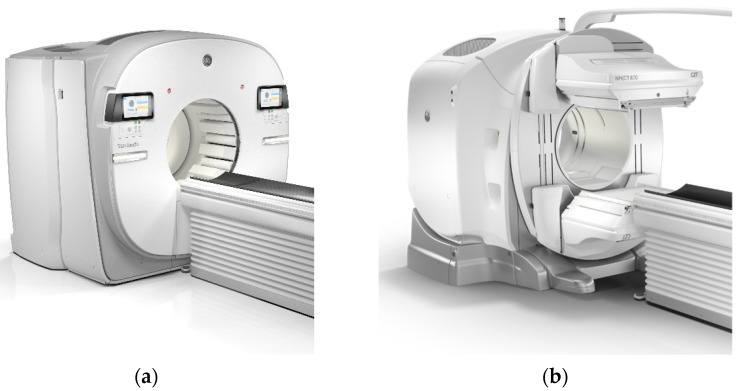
Ring-shaped SPECT: (a) StarGuide (GE Healthcare, Chicago, IL, USA) side-by-side with the conventional multi-head SPECT design, (b) NM/CT 870 (GE Healthcare, Chicago, IL, USA). Courtesy of GE Healthcare.
